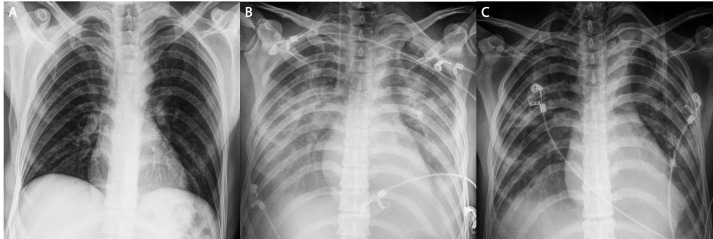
Antero-posterior chest x-rays A. Preoperative normal chest x-ray of the patient B. Postoperative chest x-ray showing extensive and diffuse bilateral pulmonary infiltrates that resulted in the diagnosis of TRALI following blood transfusions C. Postoperative chest x-ray after TRALI was resolved through a combination of adequate ventilatory support and appropriate intensive care. The chest x-ray shows a return to normal and diffuse pulmonary infiltrates are no longer detectable.
